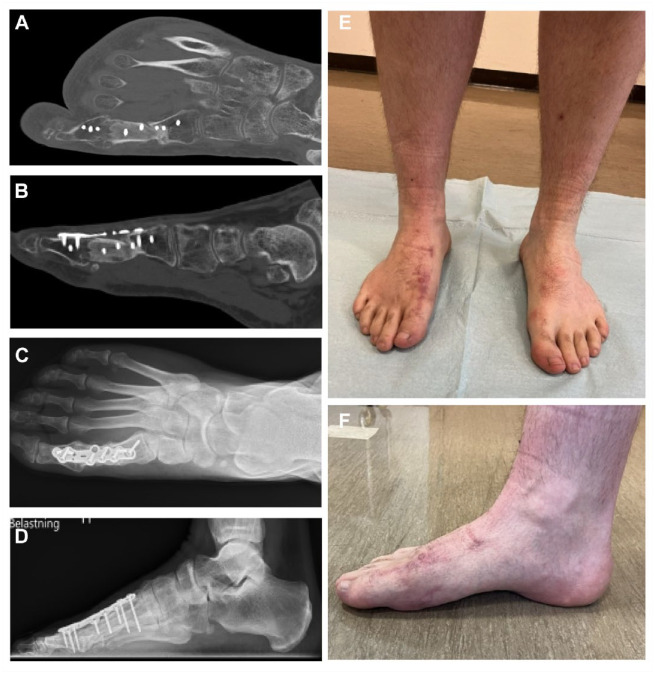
The figure shows the anteroposterior and lateral computed tomographic scans of the right foot 5 months after the graft implantation. Both the distal and the proximal part of the graft seems integrated with the proximal phalanx and the proximal end of the first metatarsal bone, even though the central part of the graft shows hyperdensity (7A and B). The anteroposterior and lateral radiographs at 16 months after graft implantation show a continuity of the first metatarsus and a fused metatarsophalangeal joint (7C and D). Figure 7E shows the anterior view of the right and left foot and Figure 7F the medial view of the right foot at 16 months after surgery.
